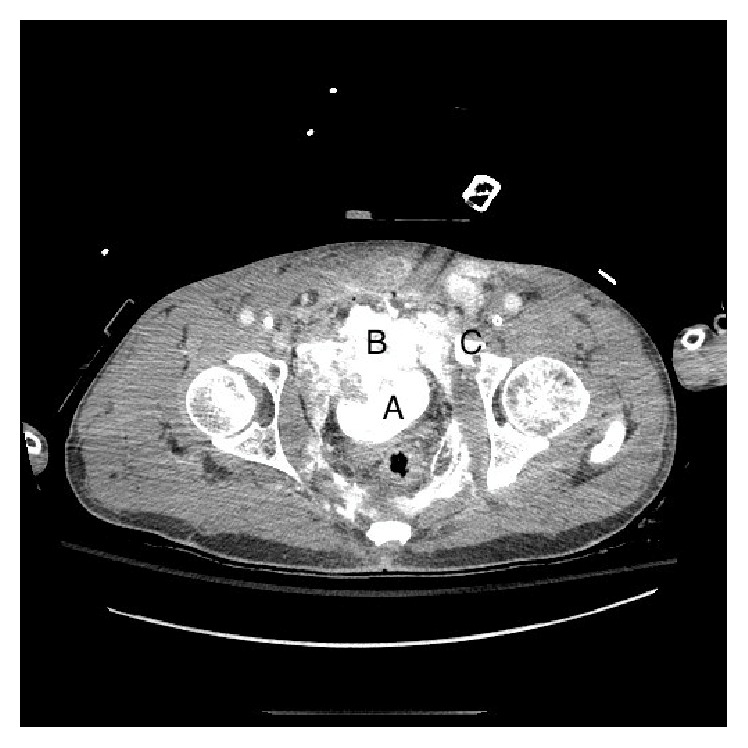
CT A/P demonstrating significant injuries in the pelvis including bladder injury with contrast extravasation and open book pelvic fractures. A: bladder filled with contrast. B: contrast extravasation anteriorly. C: fracture of the pubic ramus.
